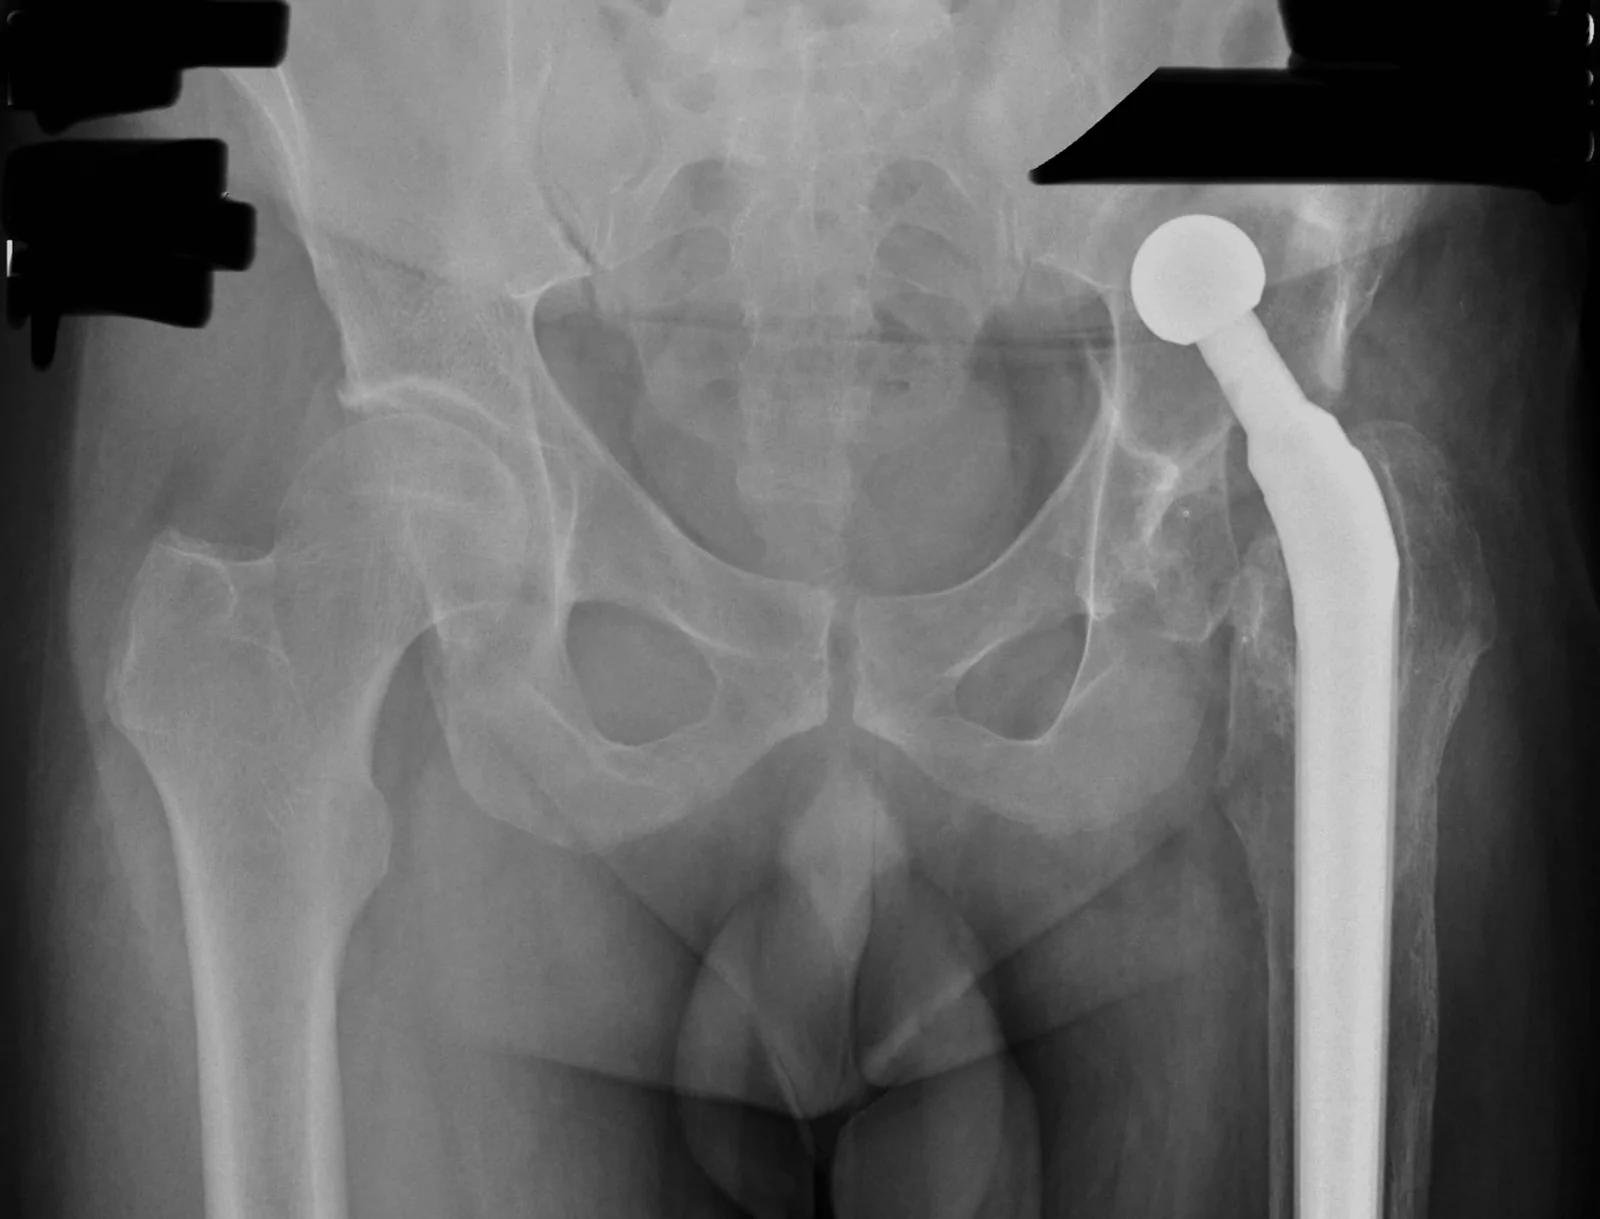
Preoperative radiographic findings. Anteroposterior pelvic radiograph demonstrating catastrophic periprosthetic osteolysis and superior-lateral migration of the left acetabular component toward the pelvic brim, accompanied by severe lateral cup inclination (62°) and marked limb shortening (2.5 cm).
